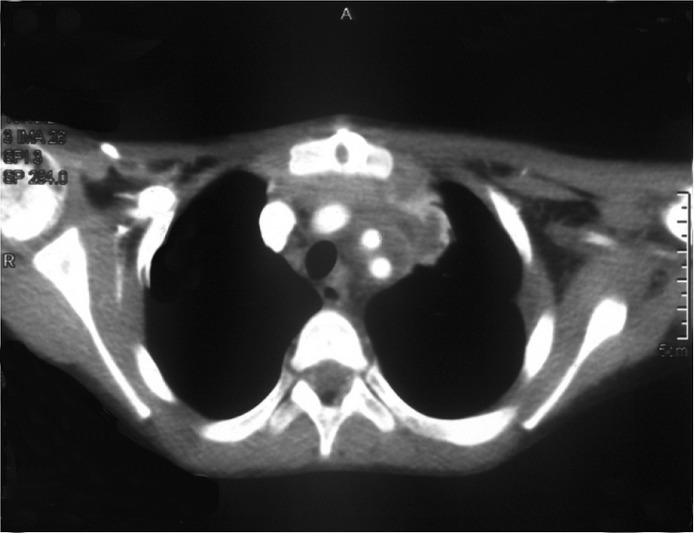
Follow-up CT subsequent to surgery showing a soft tissue mass of 5 cm in diameter found in the anterior mediastinum. CT, computed tomography.
